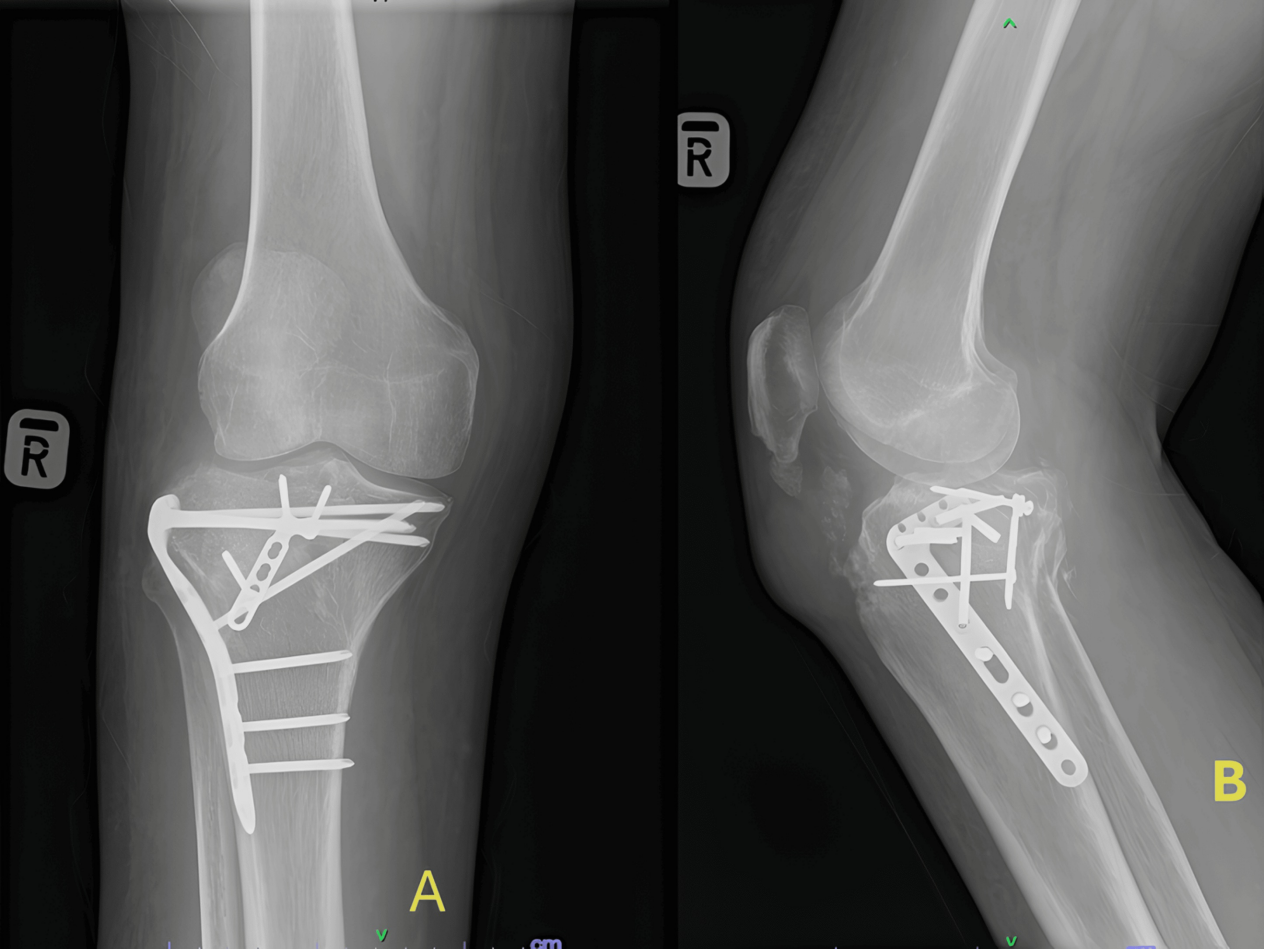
Six-month postoperative radiograph. (A) AP view showing restored congruent knee joint. (B) Lateral view showing that the posterior column is maintained with the posterior buttress AP: anteroposterior
